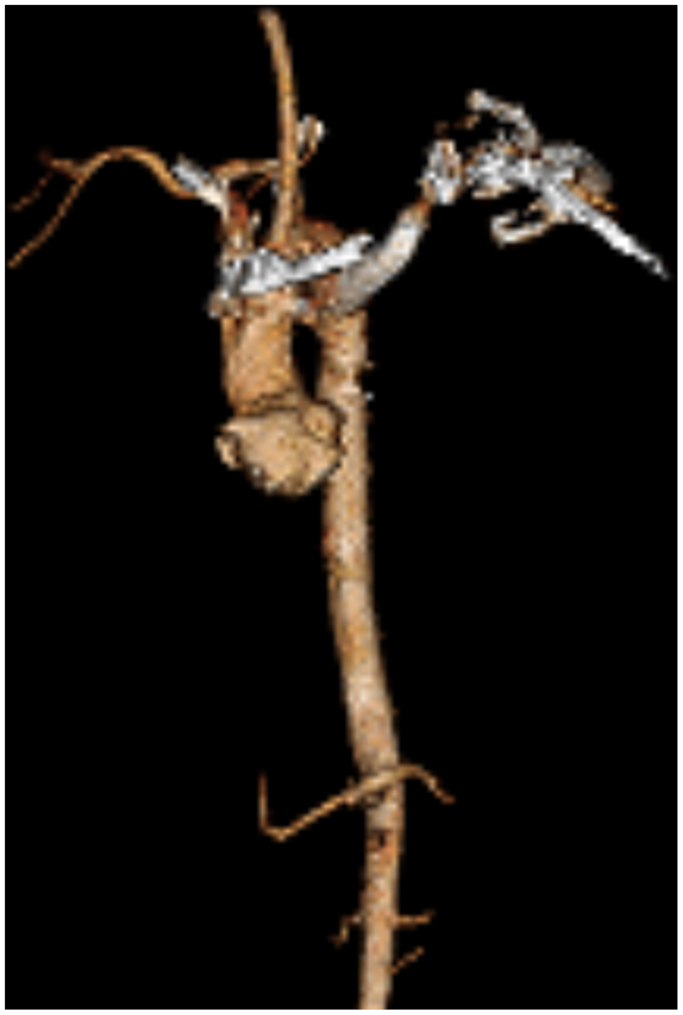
Computed tomography angiography (CTA) three-dimensional reconstruction showing the aberrant left subclavian artery/right sided aortic arch.
